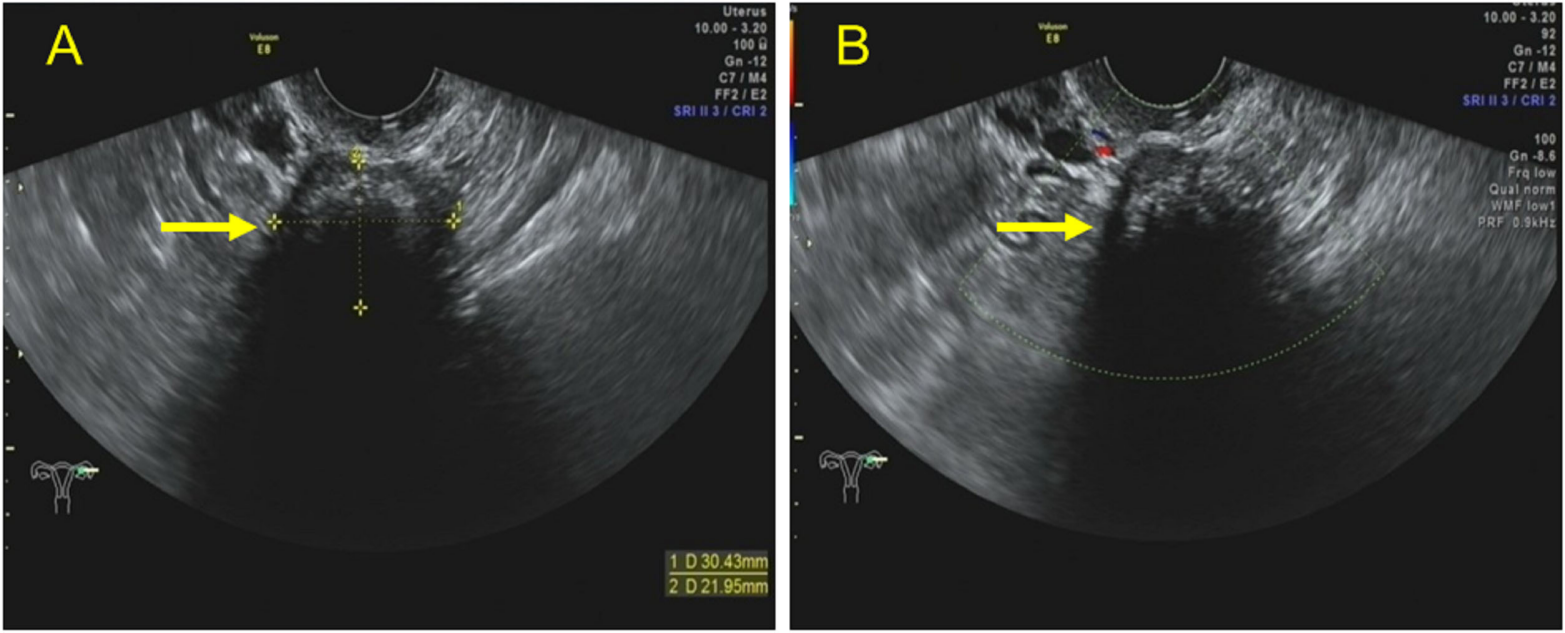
Transvaginal ultrasound images of a solid lesion with obvious calcifications in the left ovary. (A) Transvaginal ultrasound revealing a lesion with a diameter of 3.0 × 2.2 cm in the left ovary as well as arc-shaped calcifications in the nearer field and acoustic shadows in the rear. (B) Color Doppler imaging demonstrated a few flow signals in the hypoechoic part of the lesion.
